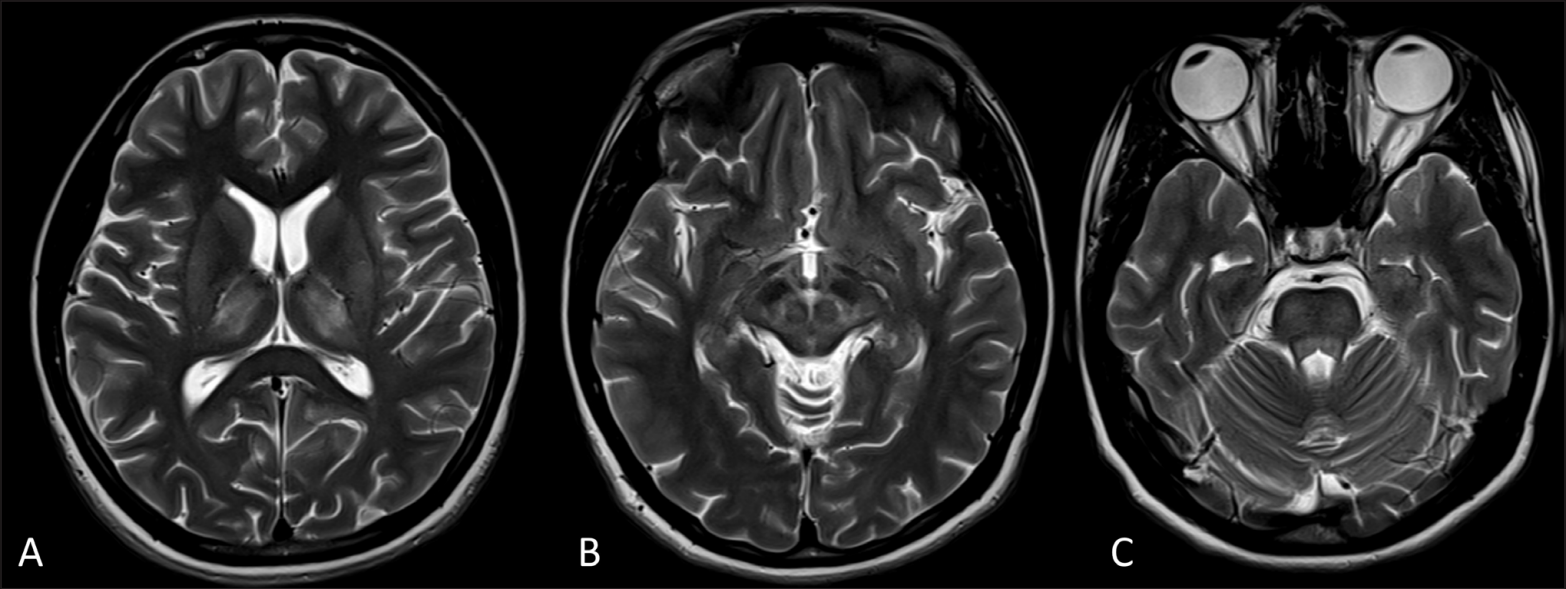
A to C- MRI axial sections of Case-1 depicting T2 hyperintensities in bilateral thalamus (A), face of the giant panda sign in the midbrain (B), and face of the miniature panda sign in the pons (C). MRI: Magnetic resonance imaging.
